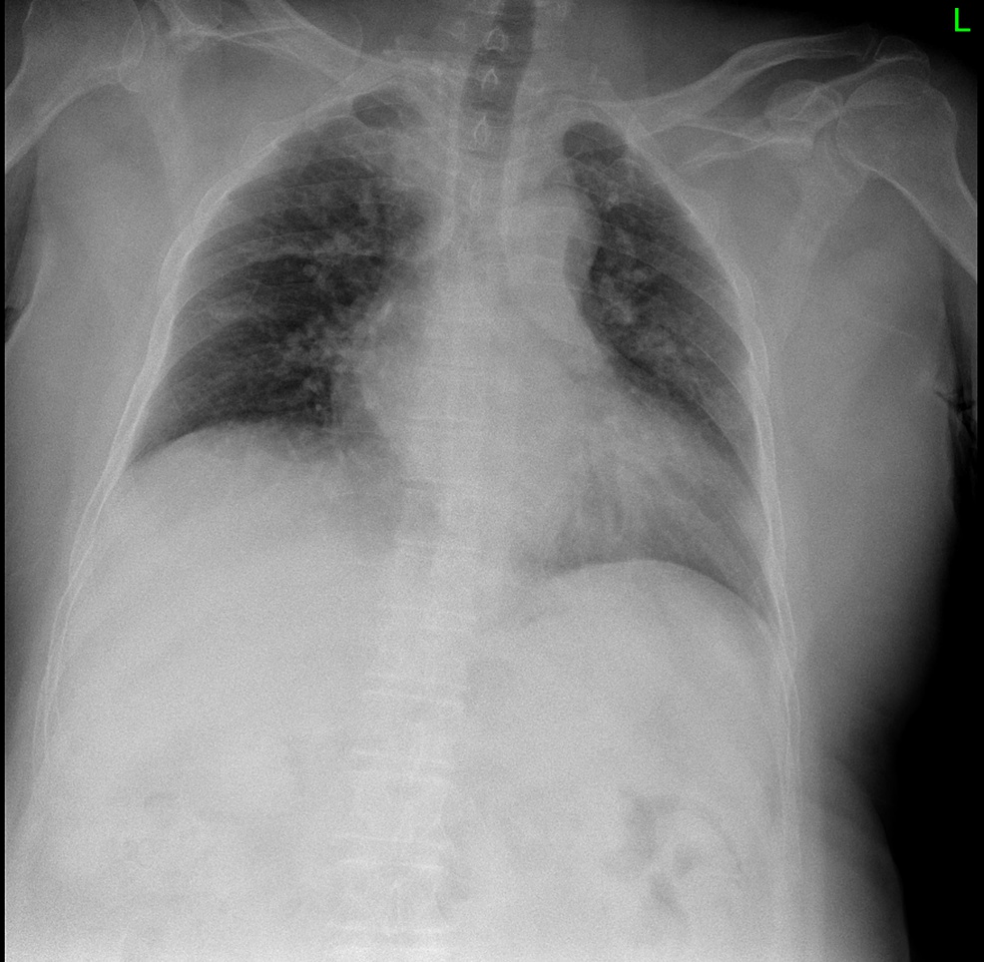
Chest X-ray showed Bilateral cephalization and left lower zone patchiness
